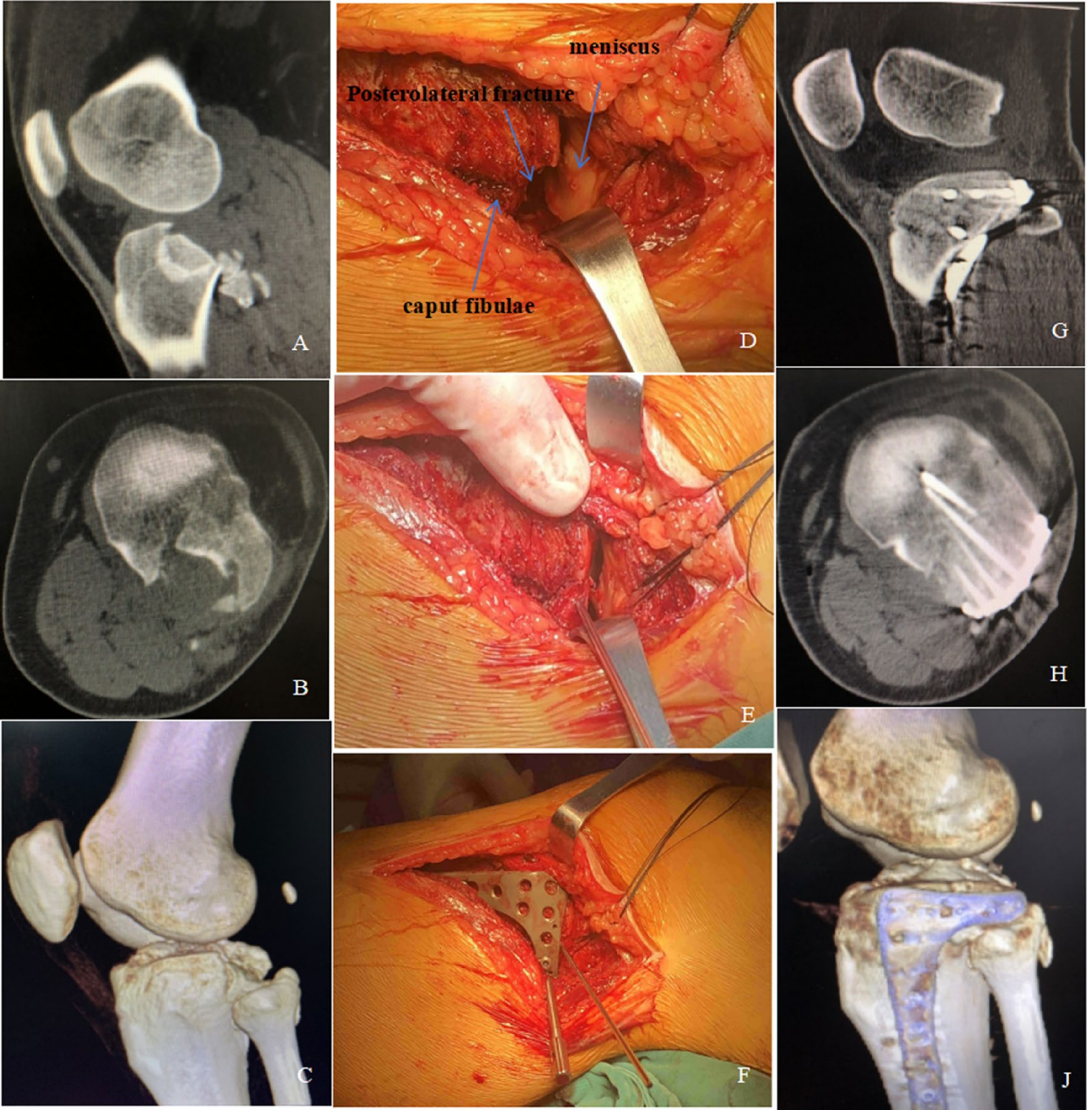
A case of type II tibial plateau fracture, male, 56 years old. (A–C) Preoperative CT showed a significantly depressed fragment in posterolateral plateau. The 3D reconstruction CT showed that the fracture involved the Area I and Area II of the lateral plateau. (D–F) Surgical exposure, reduction, and fixation of the posterolateral plateau. (G,H,J) Postoperative CT showed a satisfactory reduction of posterolateral condyle fracture, with proximal positioning of the subchondral screws (raft screws) to support posterolateral joint surface impaction injuries.
